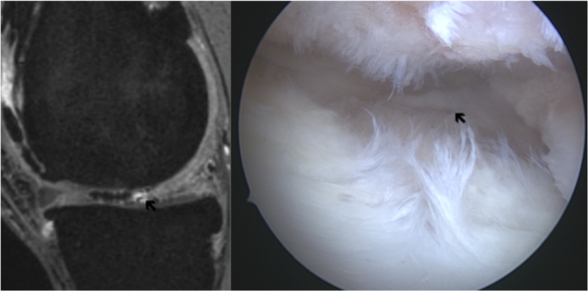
MRI and arthroscopic image of a grade III lesion in a 42-year-old male patient. The lesion is hard to miss. The cartilage remnants can be well seen and therefore a grade IV lesion can be ruled out
